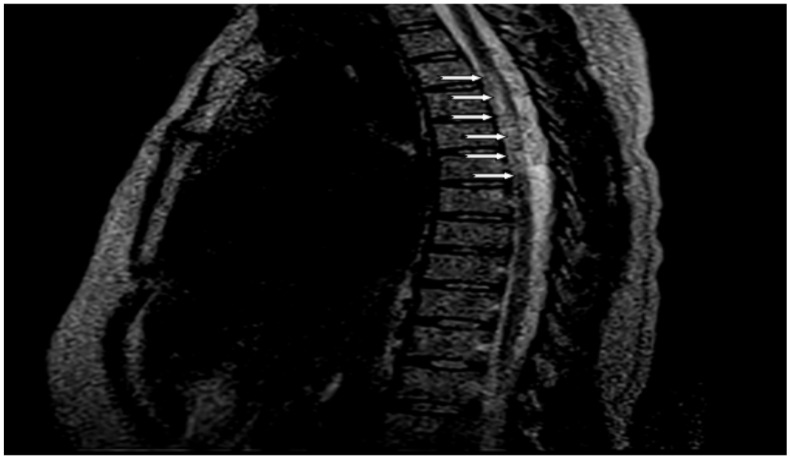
Sagittal T2-weighted Magnetic Resonance Imaging demonstrates ill-defined T2 bright signal intensities (myelitis) from T4 to T7 (white arrow)
